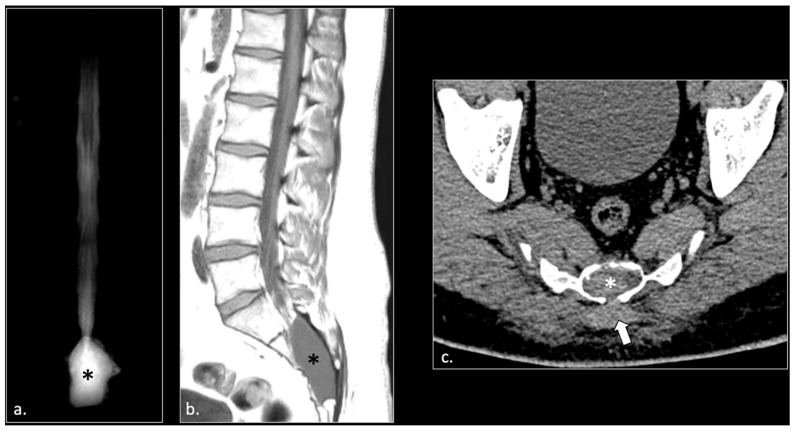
A 24-year-old male with chronic pudendalgia. The onset of pudendalgia could be referred to a sacral trauma. (a) Coronal MRI myelography shows a large Tarlov cyst in the sacral canal (*). (b) Sagittal T1 scan of the lumbar spine demonstrates slight hyperintensity of the cyst, due to chronic hemorrhage (*). (c) Computed tomography (CT scan) obtained 15 months before MRI examination, at the time of acute trauma, shows acute hemorrhage into the cyst (*) and a Morel Lavallée hematoma (arrow) in the deep subcutaneous tissue of the sacral region. Acute fracture of the sacrum around the cyst could be seen with a bone window (unshown).
